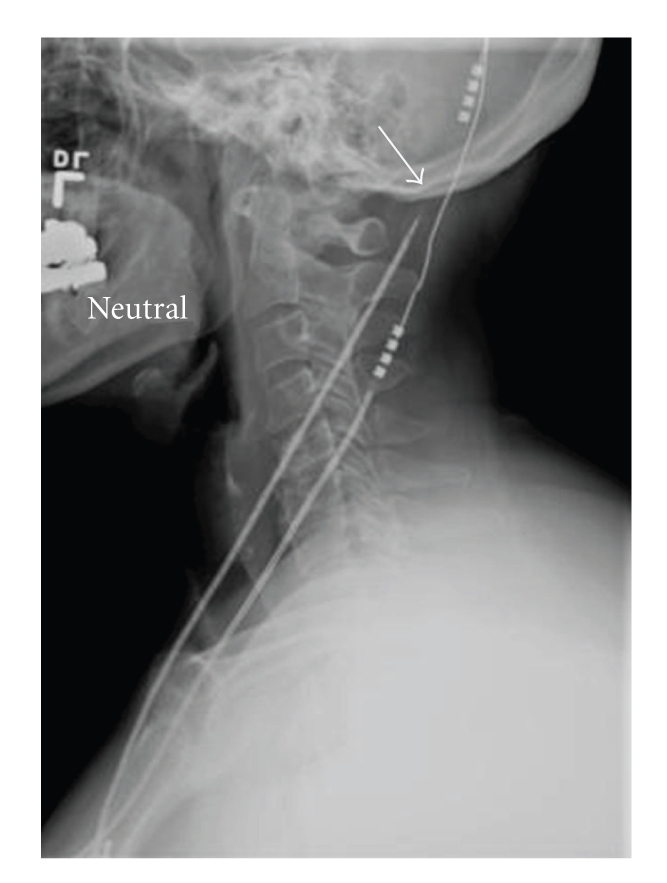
Lateral X-ray showing discontinuity of the right extension lead (arrow). Note that both the right and the left connections are low relative to the skull.
